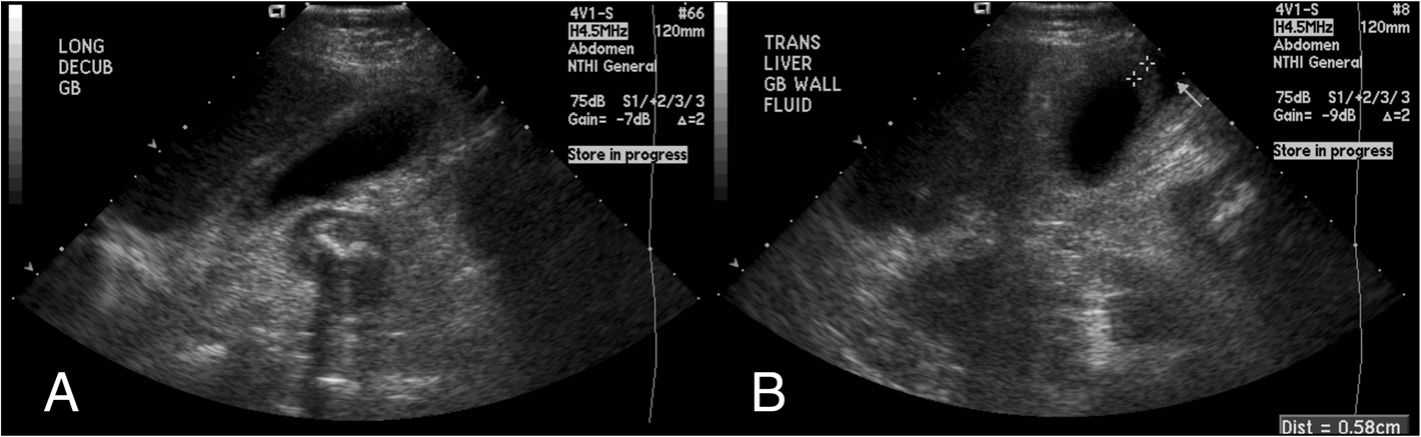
Formal right upper quadrant ultrasound #2. (A) Formal ultrasound long axis and (B) short axis views confirming gallbladder wall thickening (0.58 cm, 6 h after bedside ultrasound and initiation of diuresis).
